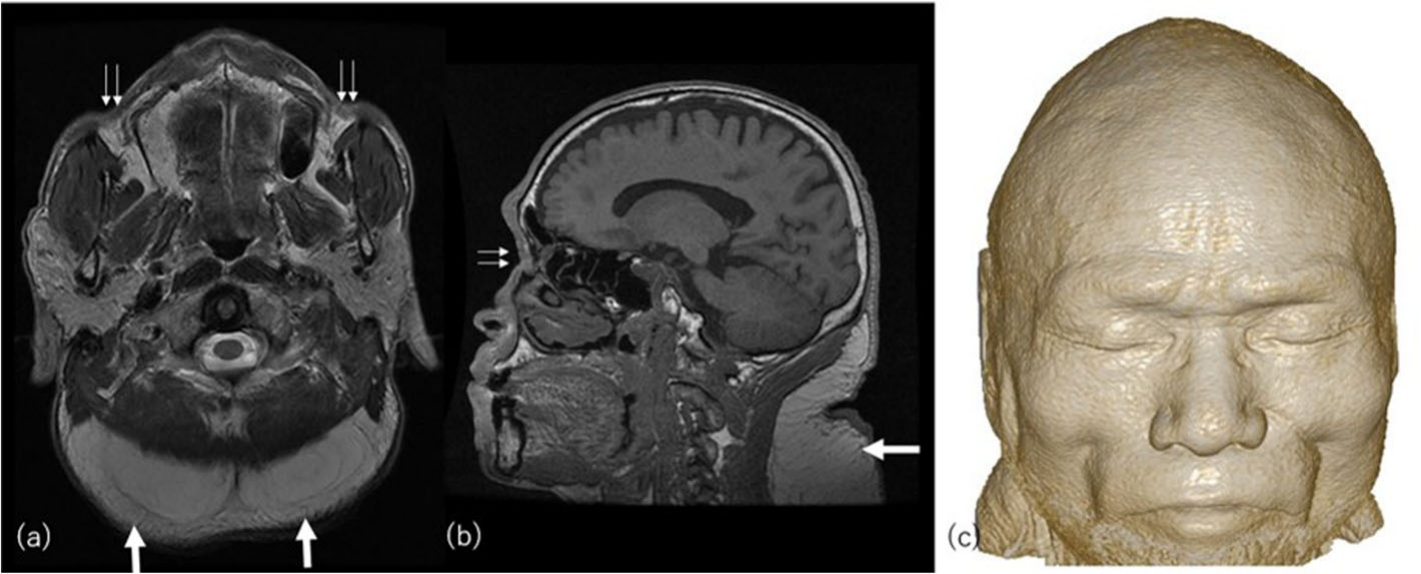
A 47-year-old man with HALS. a Axial T2-weighted and (b) sagittal T1-weighted images show subcutaneous lipohypertrophy on the posterior neck (arrows). Marked loss of subcutaneous fat including buccal fat pad is shown in the face (arrowheads). c Volume-rendered three-dimensional reformation image shows apparent concave cheeks and periorbital hollowing
